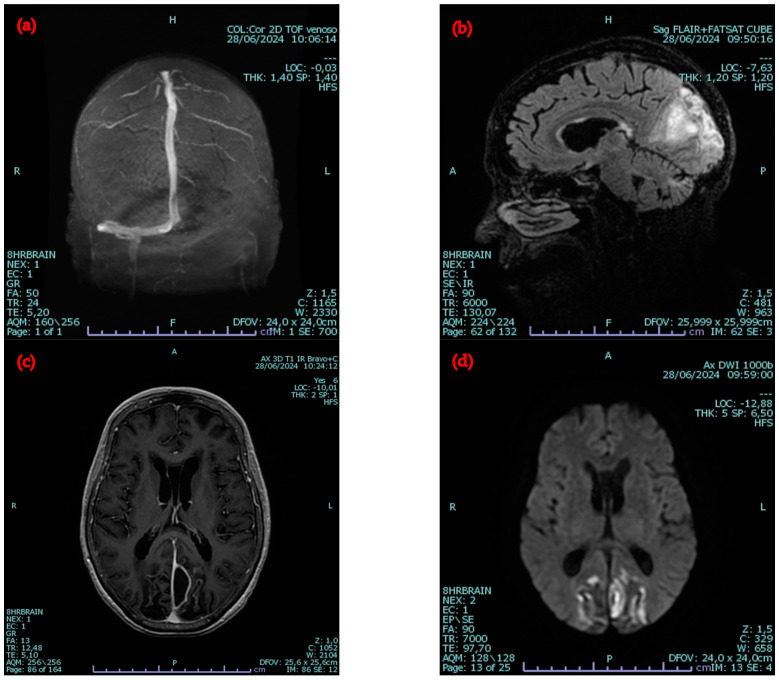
The MRI images show the following: (a) absence of flow signal in the left transverse sinus, the sigmoid sinus, and the left jugular vein in the intracranial segment, indicating thrombosis; (b) in the occipital region bilaterally, areas of altered signal compatible with sub-acute ischemic events; (c,d) an ovoid-shaped area with a maximum diameter of 28 mm, showing heterogeneous signal restriction on DWI and peripheral enhancement after contrast administration, suggestive of an abscess formation.
